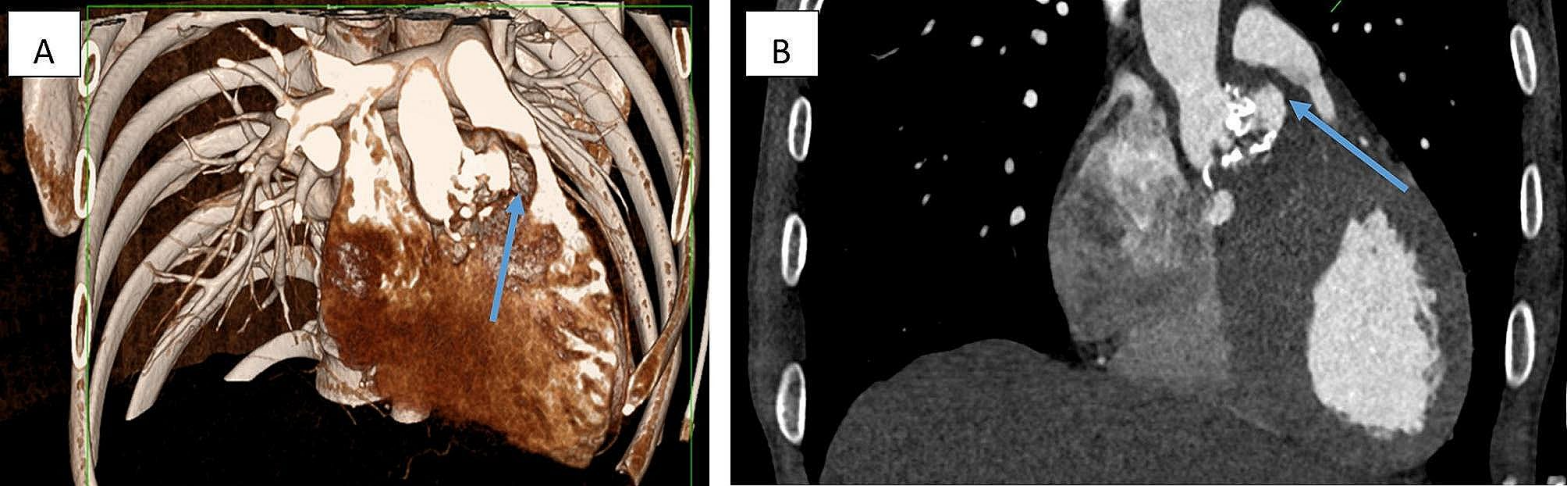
(A) 3D reconstruction showing repaired fistula, the remaining bulging was secondary to redundant patch, (B) no contrast flow was detected across the repair indicating a competent repair. This was validated by TTE doppler
